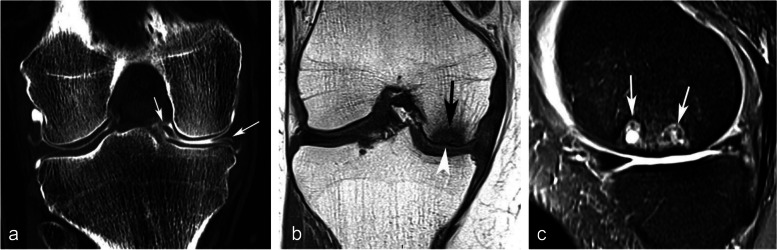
Bone decompensation after meniscectomy. a CT arthrography in a 72-year-old woman shows a horizontal tear of the medial meniscus body and a small fragment in the intercondylar notch (arrows). Ten months after meniscectomy, T1-weighted MR image (b) shows a very marked low signal intensity in the inferior pole of the medial condyle (arrow) and a small depression of the subchondral surface (arrowhead). Fat-suppressed T2-weighted image (c) shows extensive subchondral bone remodeling with heterogeneous high signal intensity in geode-like areas (arrows)
